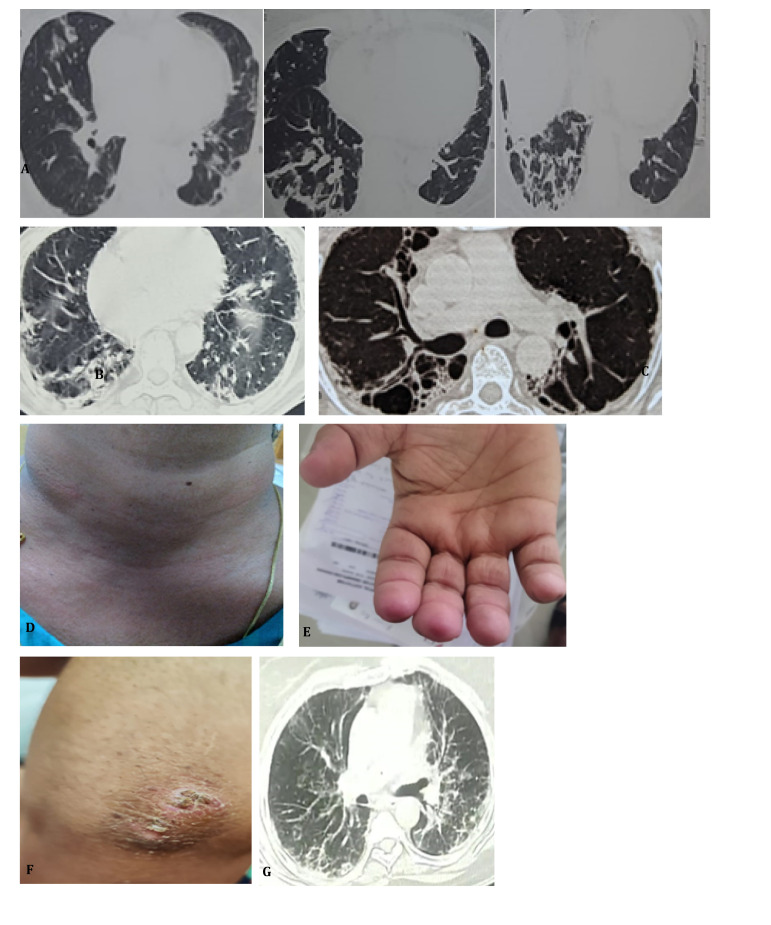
Clinical and radiological images of patients with anti-MDA 5 related RP-ILD. (A) High resolution CT chest axial images of case 1 showing bilateral intralobular septal thickening and traction bronchiolectasis and bronchiectasis with basal predominance. (B) High resolution CT chest axial images of case 2 showing ground glass opacities and dense septal inflammation with traction bronchiolectasis which progressed to fibrosis and nonspecific reparative cysts and traction bronchiectasis (C). Erythematous rash on anterior aspect of neck suggestive of V sign in Case 3 (D) and palmar papules at the tip of index and middle finger of right hand in Case 4 (E). Gottron’s papules over the extensor side of right elbow (F) and high-resolution CT chest axial images showing bilateral interstitial infiltrates, ground glass opacities and septal thickening in bilateral lower lobes in Case 5 (G).
